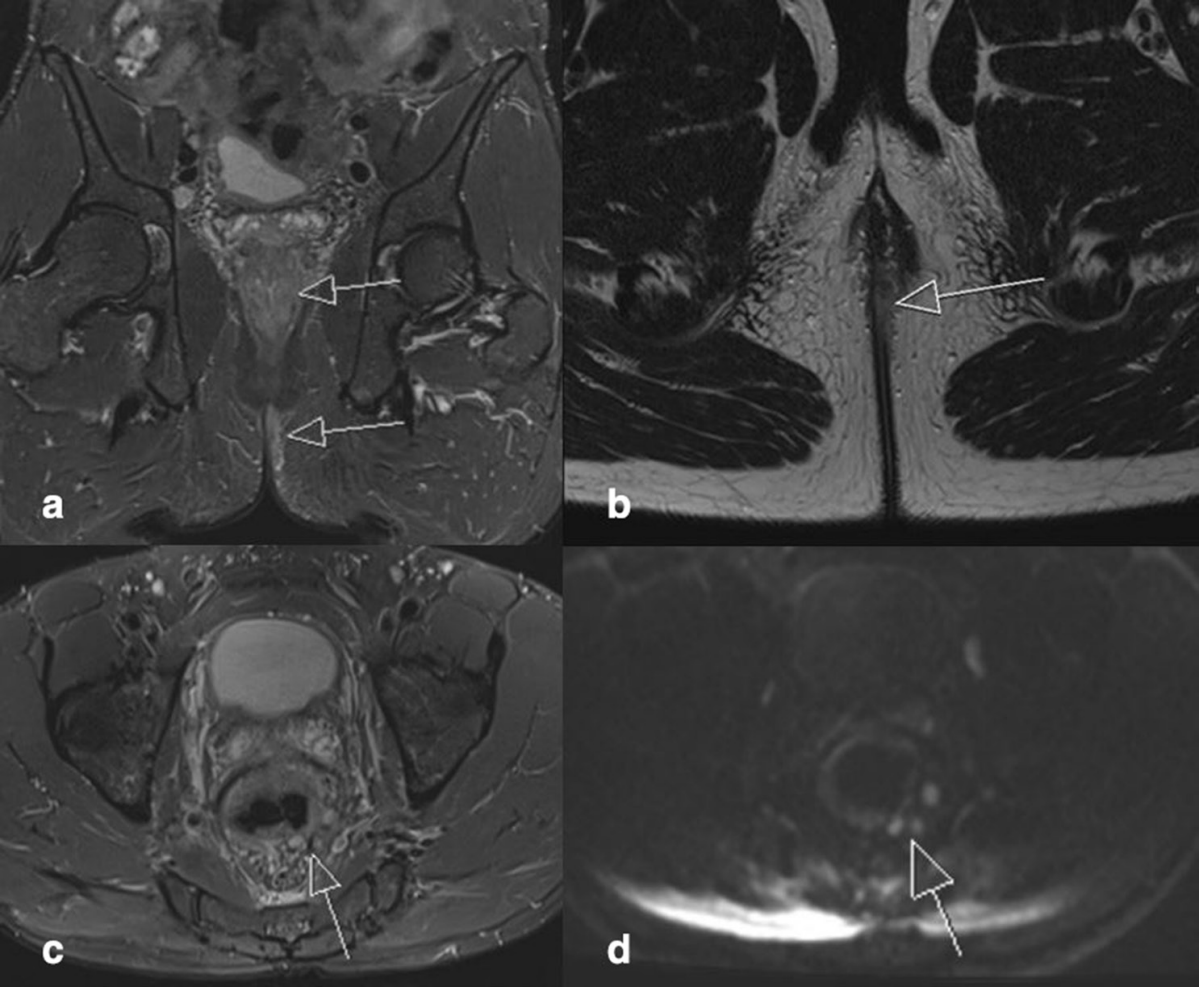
A. Coronal STIR image demonstrates high signal and mural thickening of the rectal wall with surrounding inflammation and mesorectal lymphadenopathy in keeping with severe active proctitis (superior arrow). There is also high signal in the perianal/perineal region in keeping with active inflammation of the left perianal region (inferior arrow). Figure 3B Axial T2 image demonstrates perineal/perianal active inflammation (arrow) Figure 3C. Axial STIR image demonstrates prominent mesorectal lymph nodes secondary to the adjacent proctitis. Figure 3D. Axial DWI image demonstrates corresponding restricted diffusion in the mesorectal lymph nodes.
